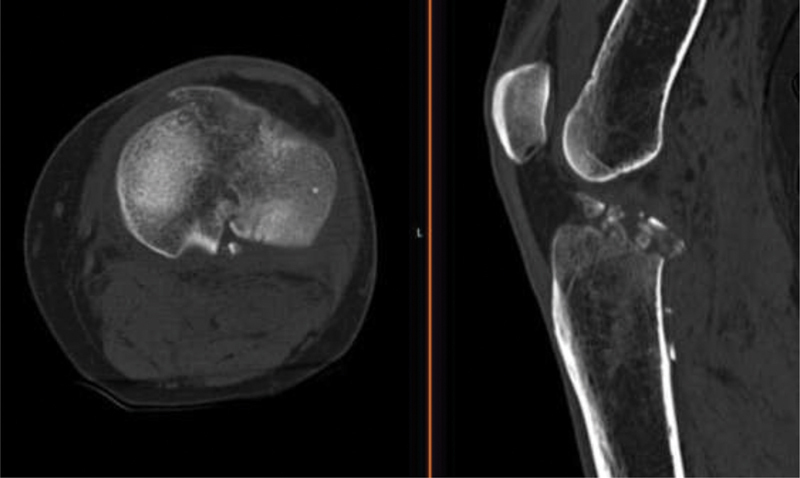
Axial and sagittal cuts of the knee computed tomography scan.
